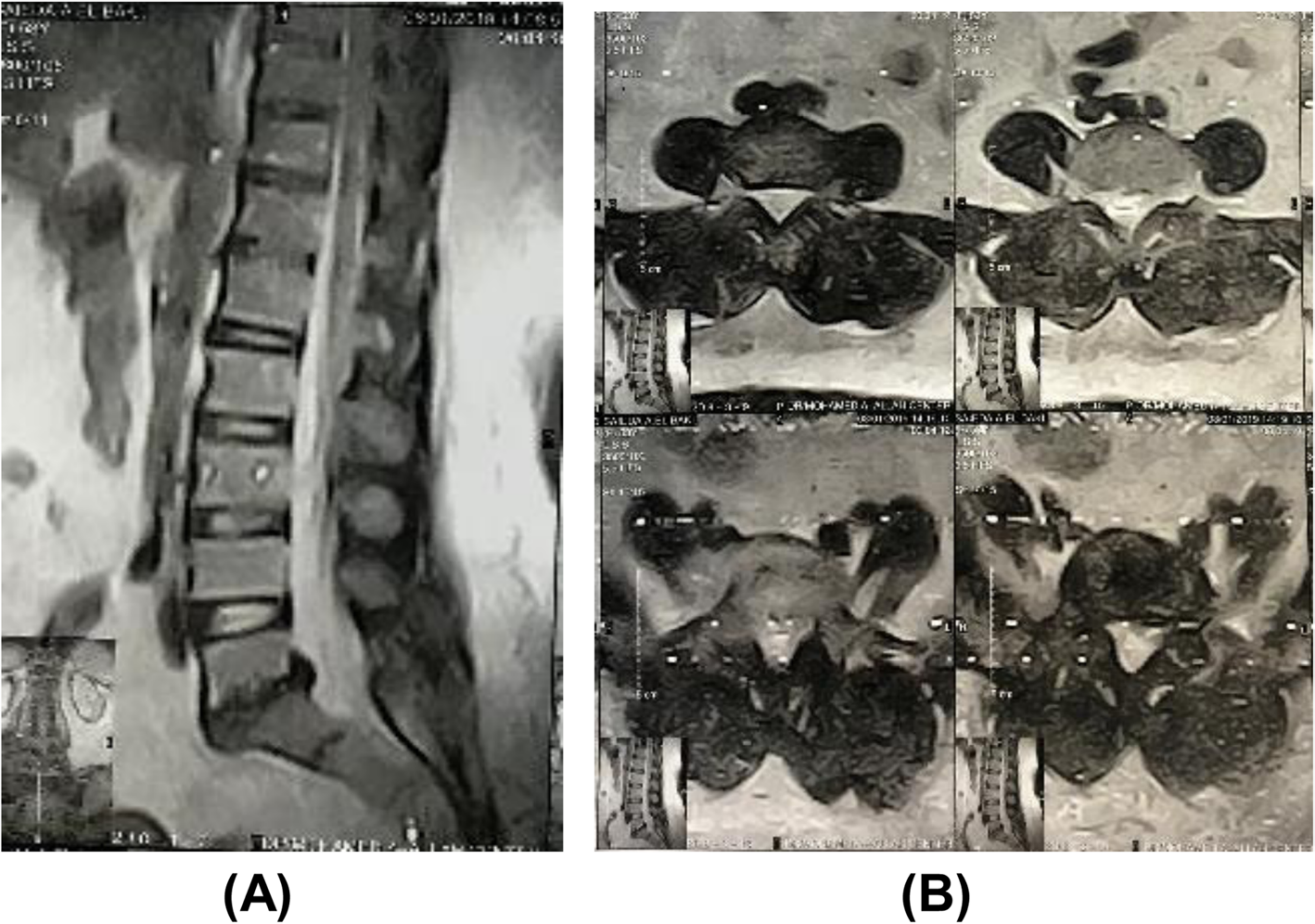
Preoperative MRI scan of the patient A sagittal and B axial cuts showing lumbar disc prolapse of L5S1 with low-grade spondylolisthesis grade 1
